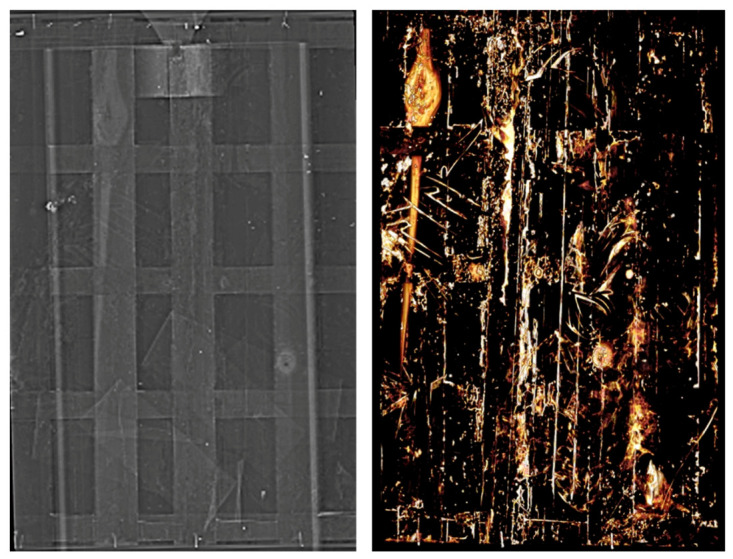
CT scan. (Left) wide bandage folded several times in a zig zag and a trapezoidal wooden insert with a hook; (right) Greek letters.
